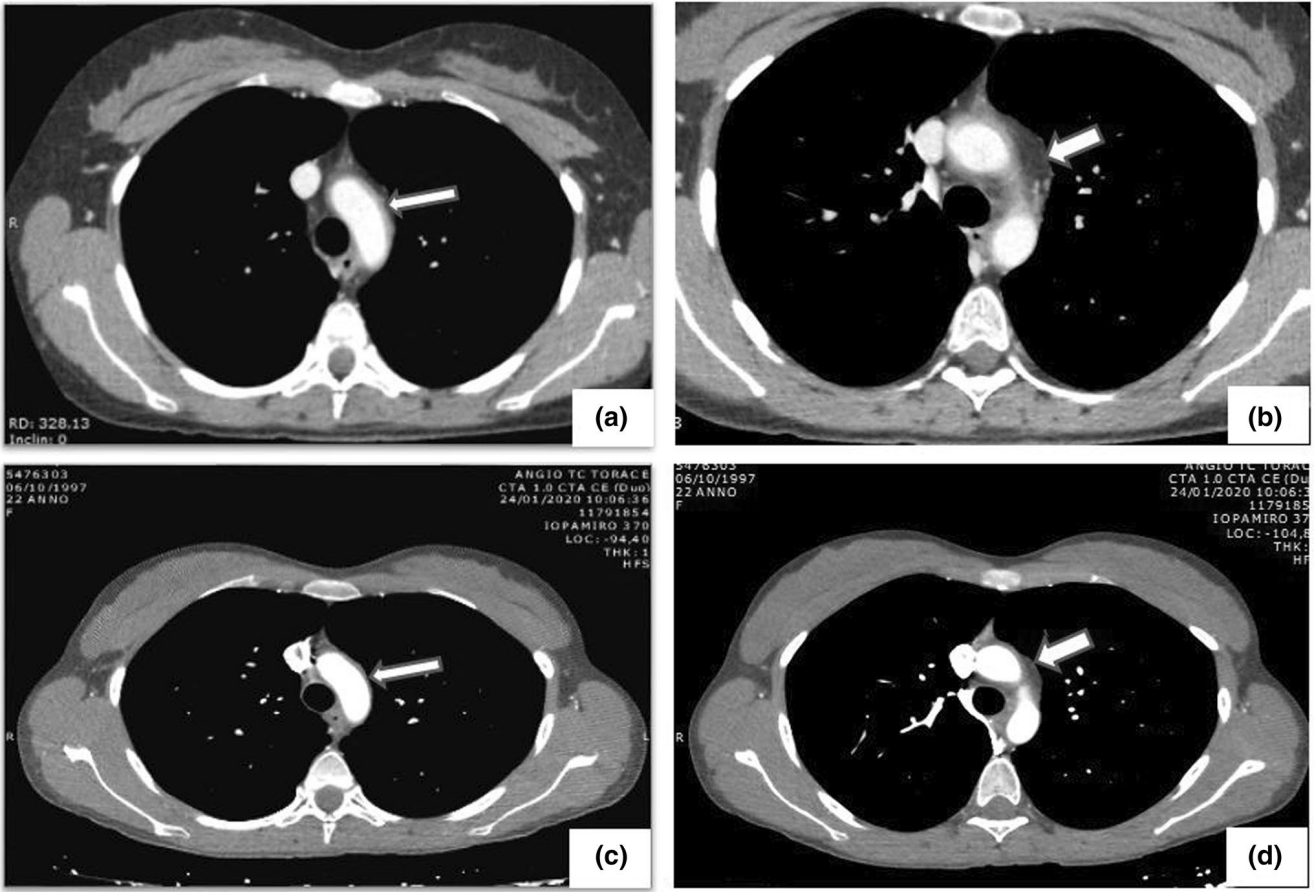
a, b Angio-CT with intravenous iodinated contrast medium: note the circumferential thickening and enhancement of the aortic arch wall (arrows). c, d Following medical treatment and using the same sequence scans, both wall thickening and enhancement are clearly reduced
